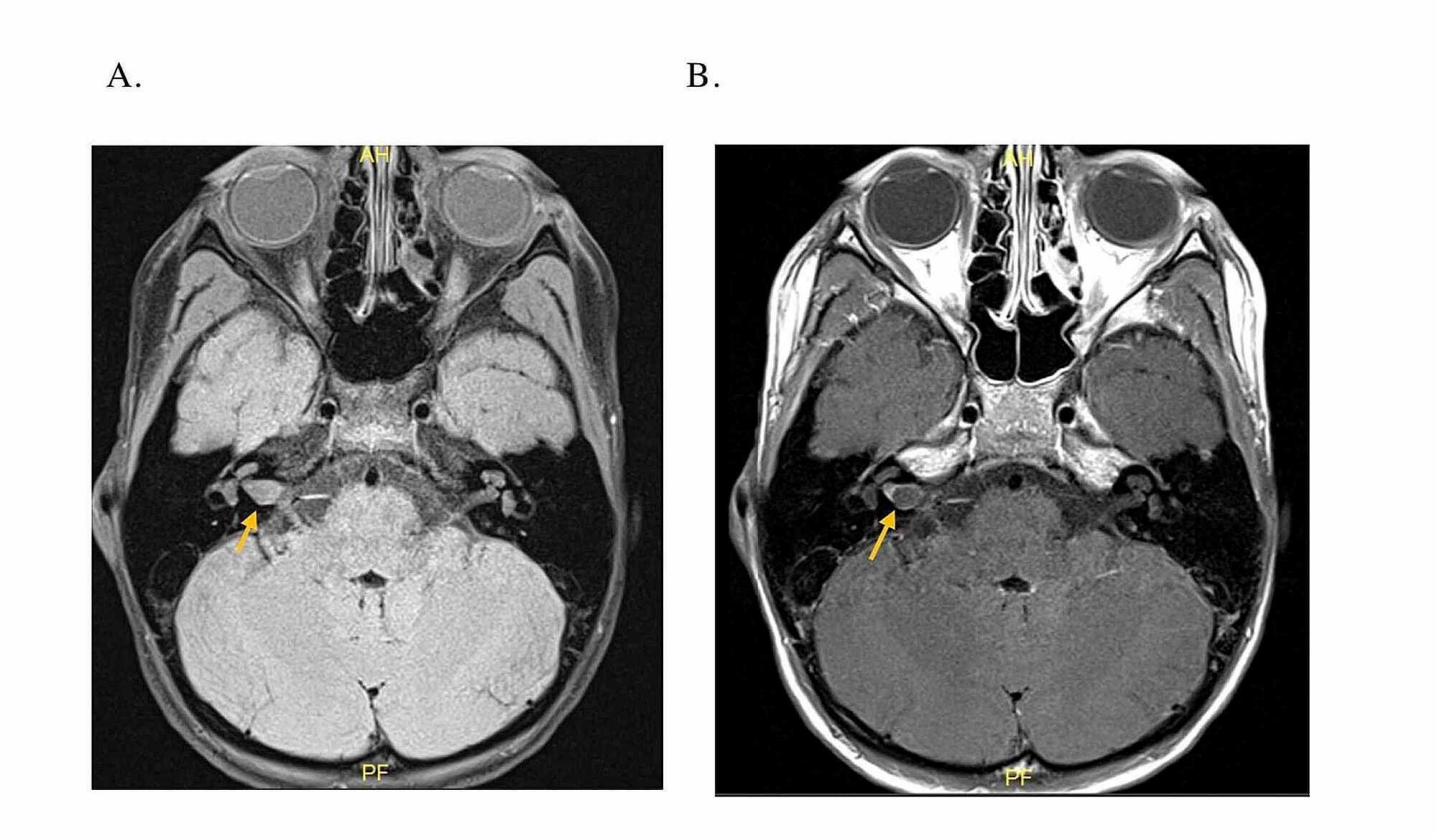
High-resolution MRI of the IAC in the axial plane. A. Demonstrates a T1 sequence without contrast showing mild asymmetric enlargement of the right IAC secondary to a cystic mass. B. T1 post-contrast showing a non-enhancing cystic mass with a fluid level in the right IAC. IAC, internal auditory canal
